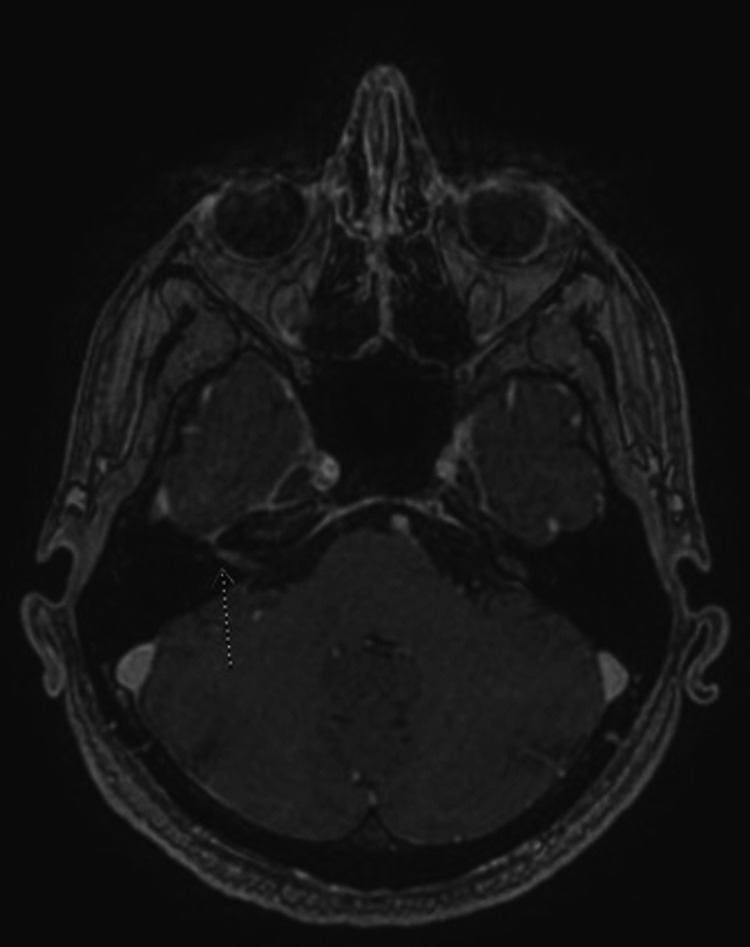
Magnetic resonance imaging (MRI) of the brain Arrow shows asymmetric enhancement of the right facial nerve
